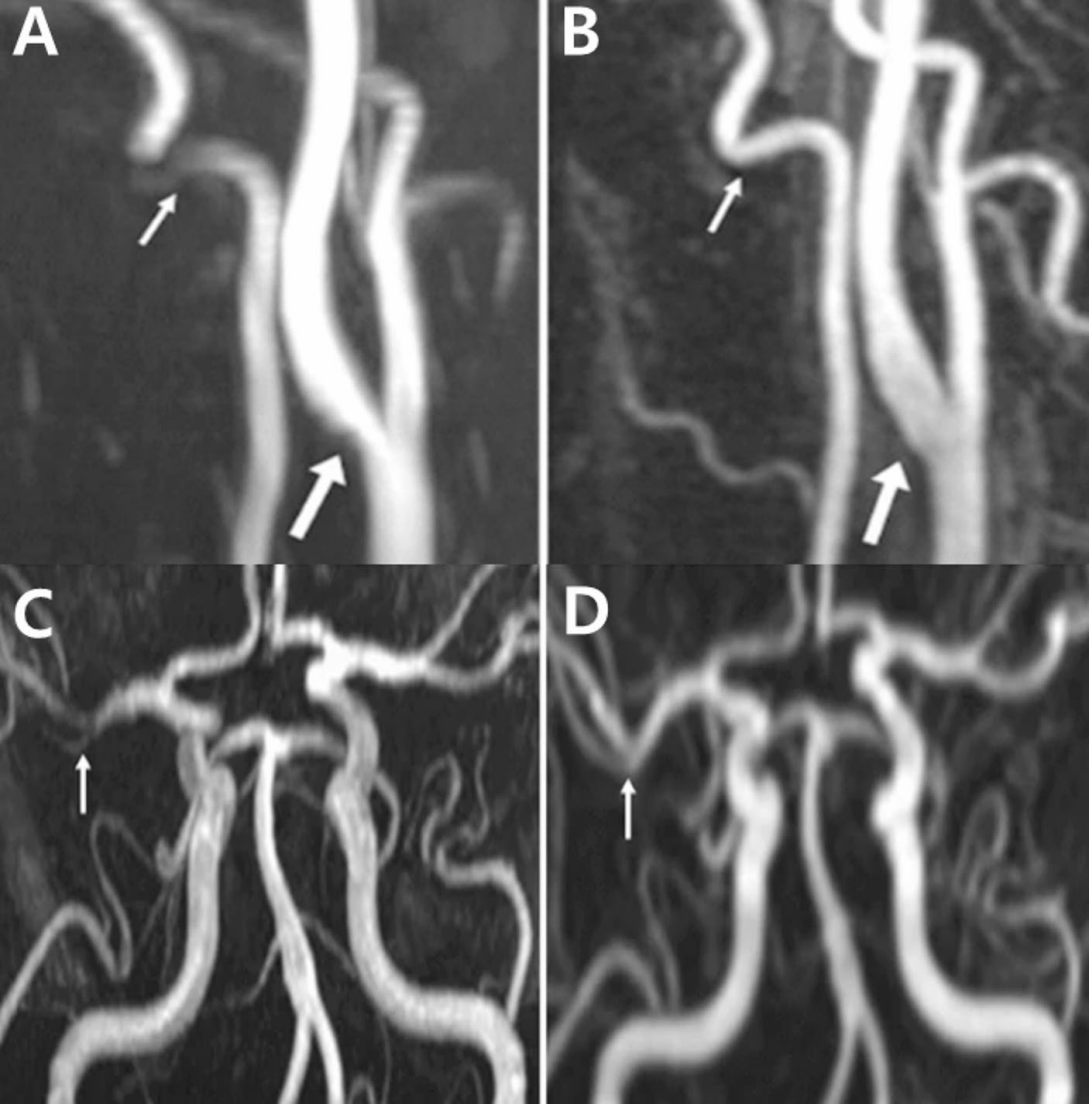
In-plane saturation artifact. (A) Pseudo-stenosis in the ICA (thick arrow) and in-plane saturation artifacts in the VA (thin arrow) are observed in a 2D TOF MRA. (B) The contrast-enhanced MRA shows normal findings in the previously observed areas. (C) A pseudo-stenosis that appears as an artificial defect in the right MCA (arrow) is evident on a 3D TOF MRA. (D) However, this defect is not seen in the contrast-enhanced MRA image. Figure adapted from McKinney AM: Artifacts of the Craniocervical Arterial System on MRI. In: *Atlas of Normal Imaging Variations of the Brain*,* Skull*,* and Craniocervical Vasculature*. Cham: Springer International Publishing; 2017: 1261–1291, under the terms of the Creative Commons Attribution License [16]
